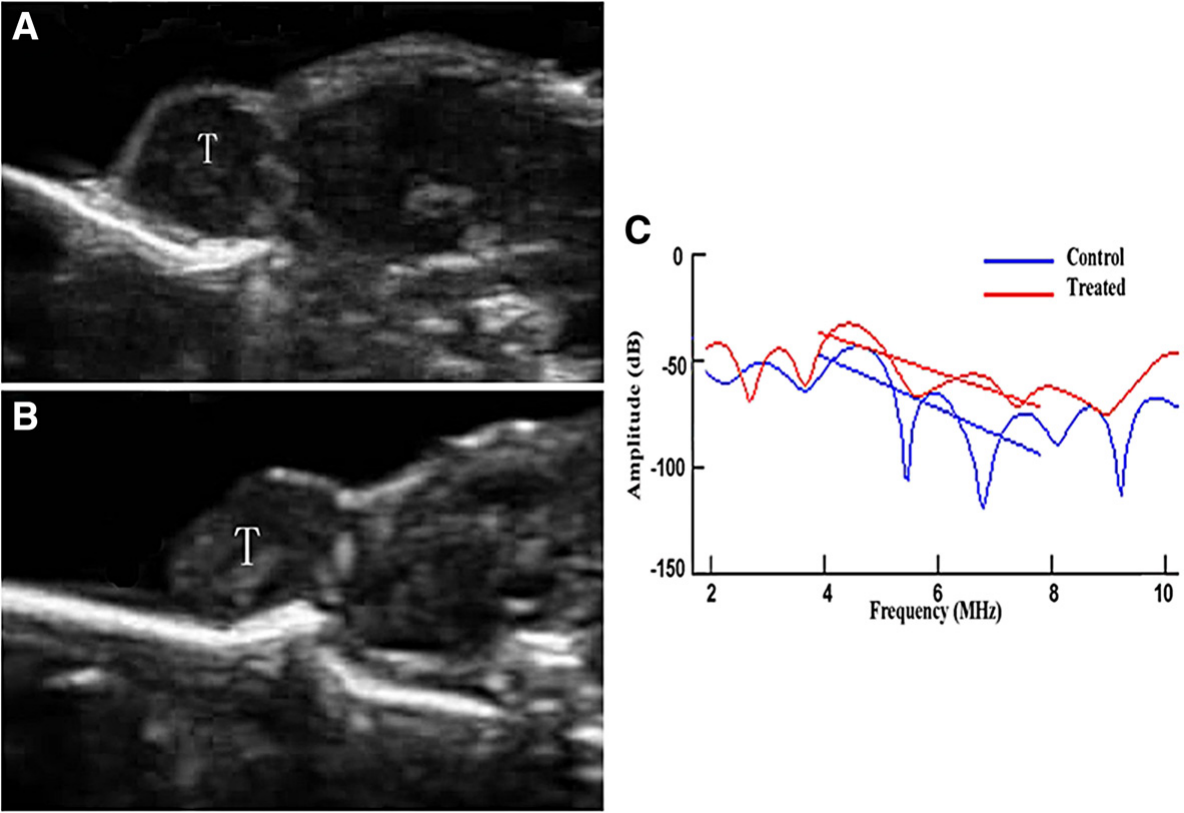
Representative ultrasound images and corresponding ultrasound spectral parameters. Ultrasound images for (A) a control tumor and (B) a treated tumor, and the corresponding ultrasound spectral parameter (C) characterization of tumor responses to chemotherapy. The ultrasound images showed a noticeably brighter grayscale intensity in treated tumors (B) relative to control tumors (A). (C) Ultrasonic spectral analysis indicated a separation of the regression line between the control and the treated tumors.
